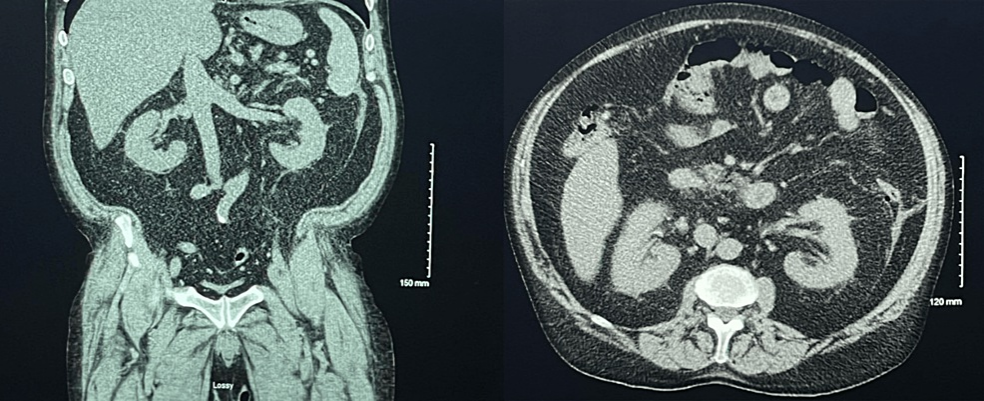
CT scan of the abdomen and pelvis Computed tomography (CT) scan of the abdomen and pelvis demonstrated normal morphology of the kidneys and liver. Left-hand side image: Coronal view, Right-hand side image: Axial view
